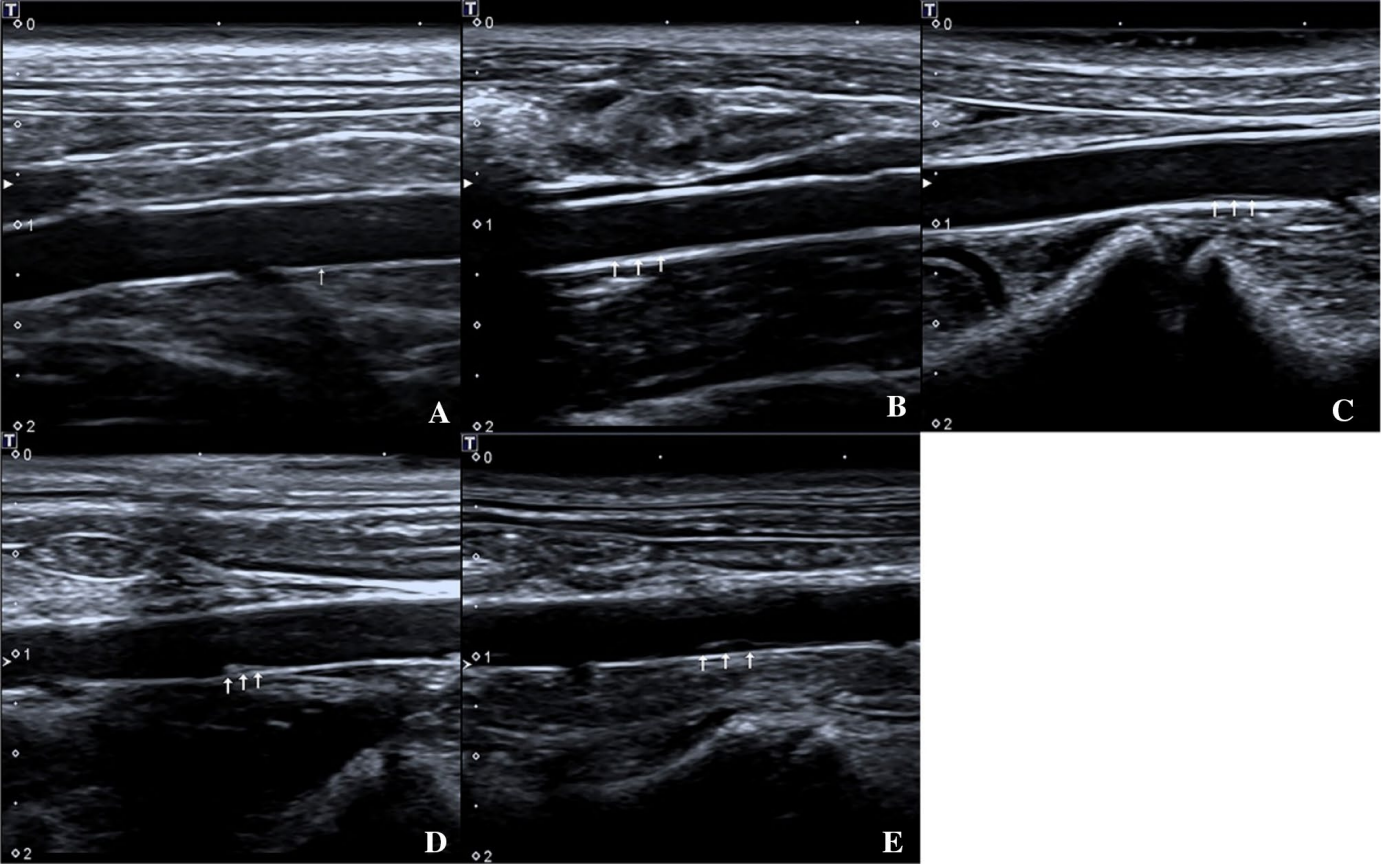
Two-dimensional ultrasonography images of the abdominal aorta of rabbits in different groups, five rabbits in each group. (A) Control group; (B) Week-4 group; (C) Week-8 group; (D) Week-12 group; (E) Week-16 group. The intima-media membrane and the plaque on the wall was indicated by the arrows.
